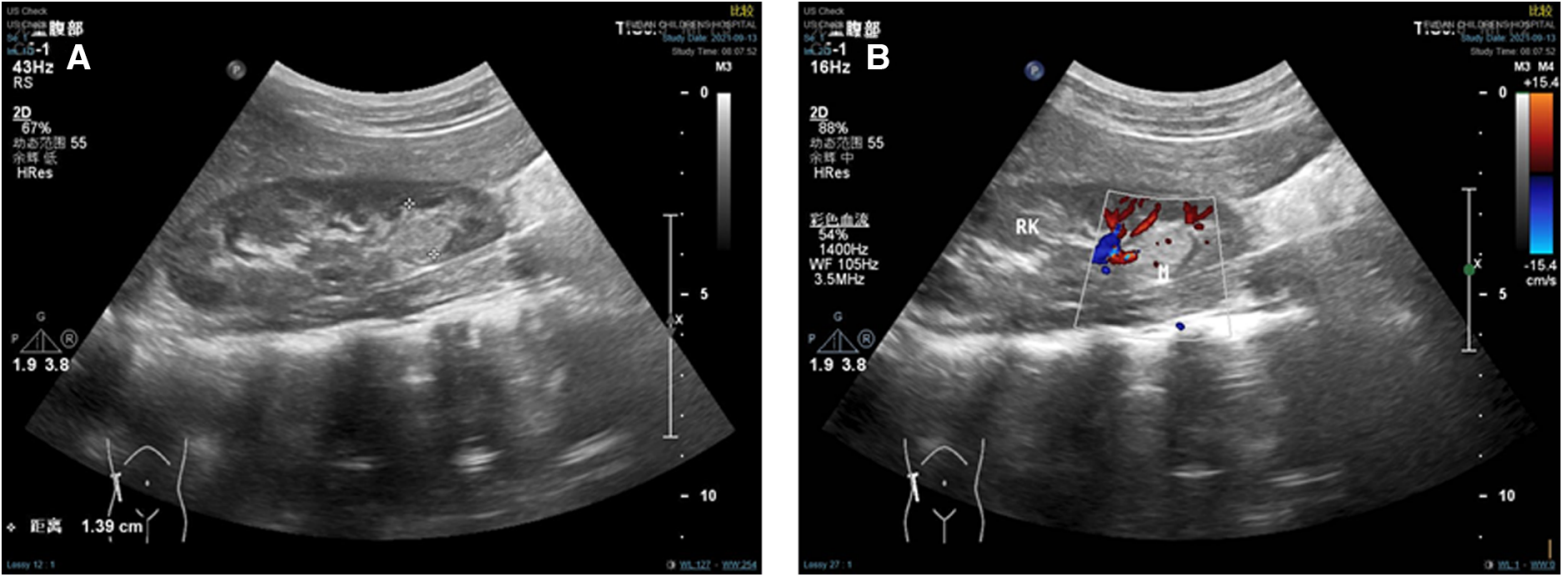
Preoperative ultrasound results. (A) Ultrasound showed a moderate echogenic area at the dorsal side of the lower pole of the right kidney. (B) Ultrasound showed blood flow signal inside the echogenicity.
